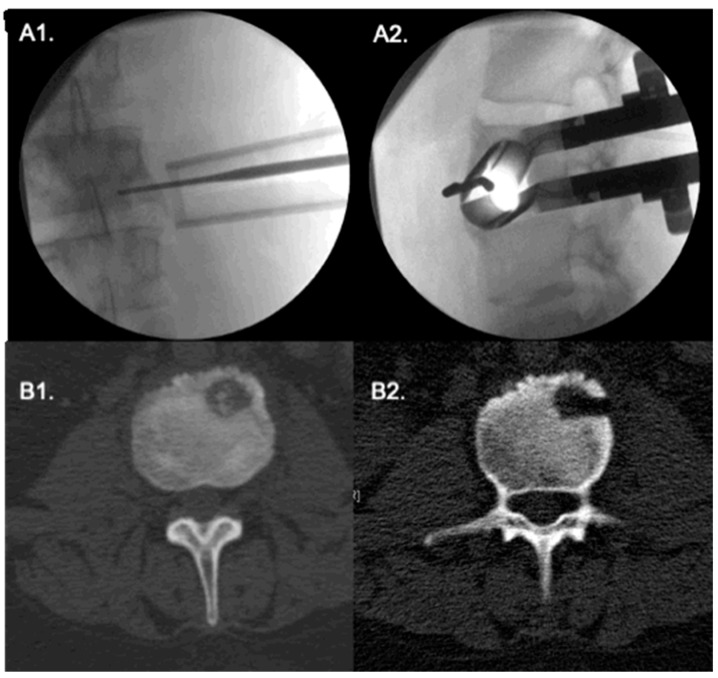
Extreme lateral approach to an L3 vertebral body tumor. (A1,A2) Antero-posterior and lateral fluroscopy show the correct positioning of tubular retractor; the probe points to the tumor. (B1,B2) intraoperative CT show the tumor. A pituitary forceps is shown inside the lesion.
